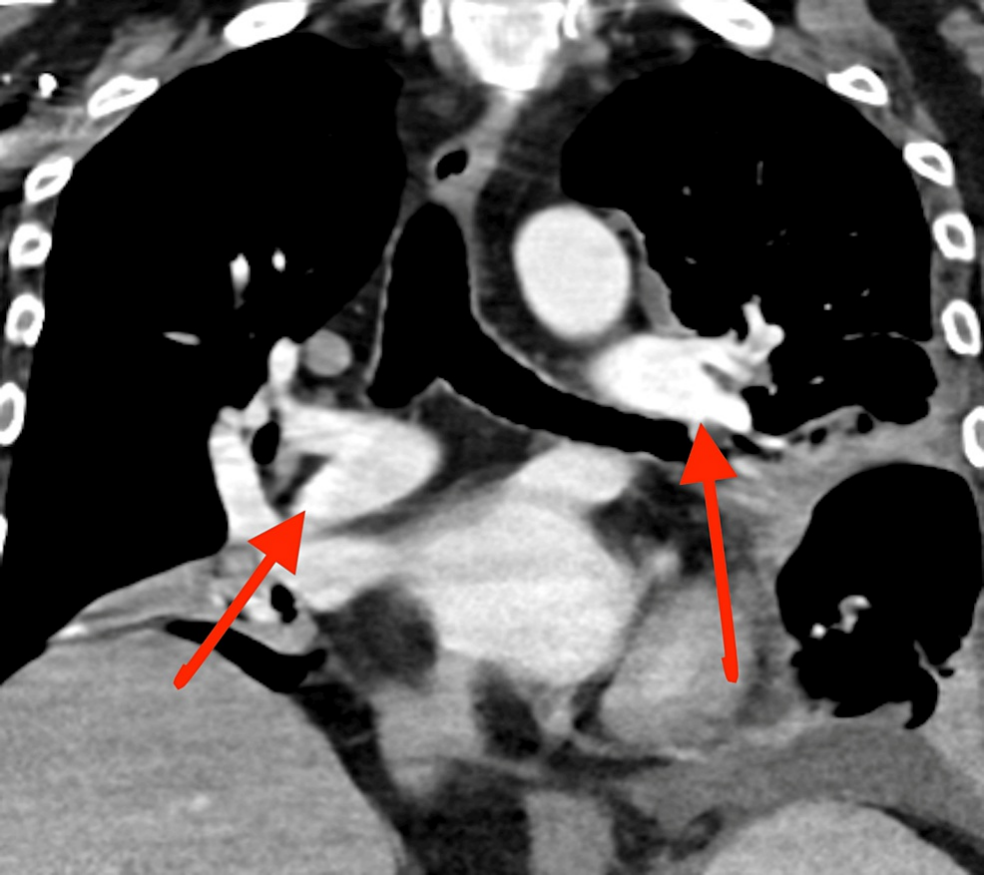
Repeat CTA of the chest one month following thrombectomy demonstrated resolution of previously identified pulmonary clot burdens (red arrows)
